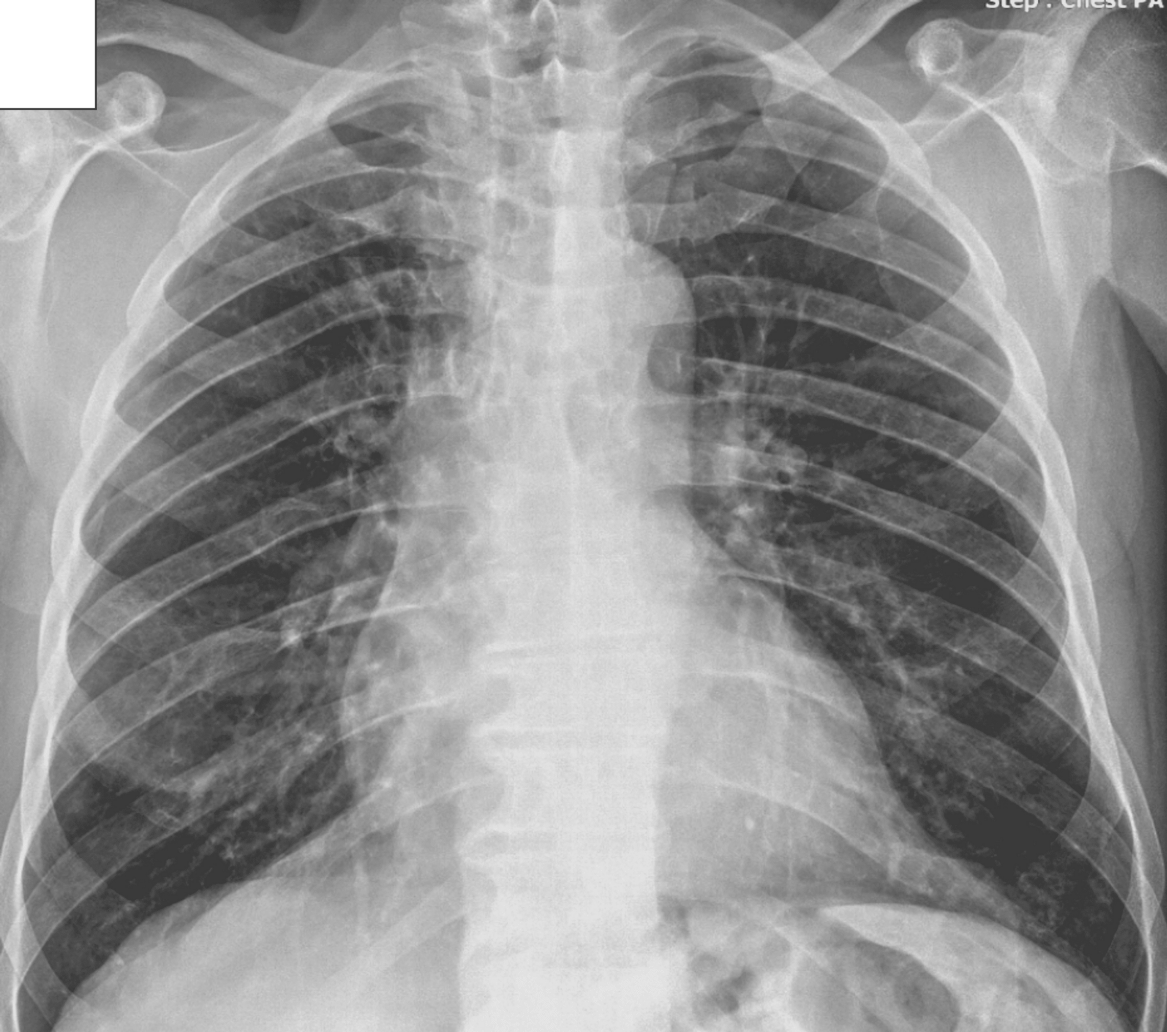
Follow-Up Chest X-Ray After ABPA Treatment ABPA, Allergic bronchopulmonary aspergillosis
